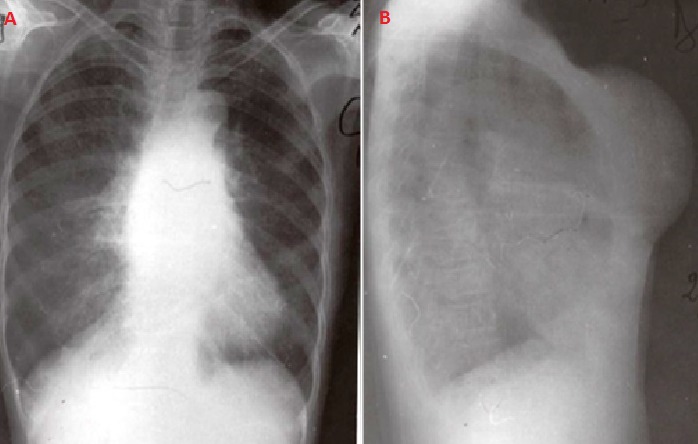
Radiographie du thorax en incidence de face (A) et profil (B) montrant une opacité pré-sternale avec lyse osseuse sternale
